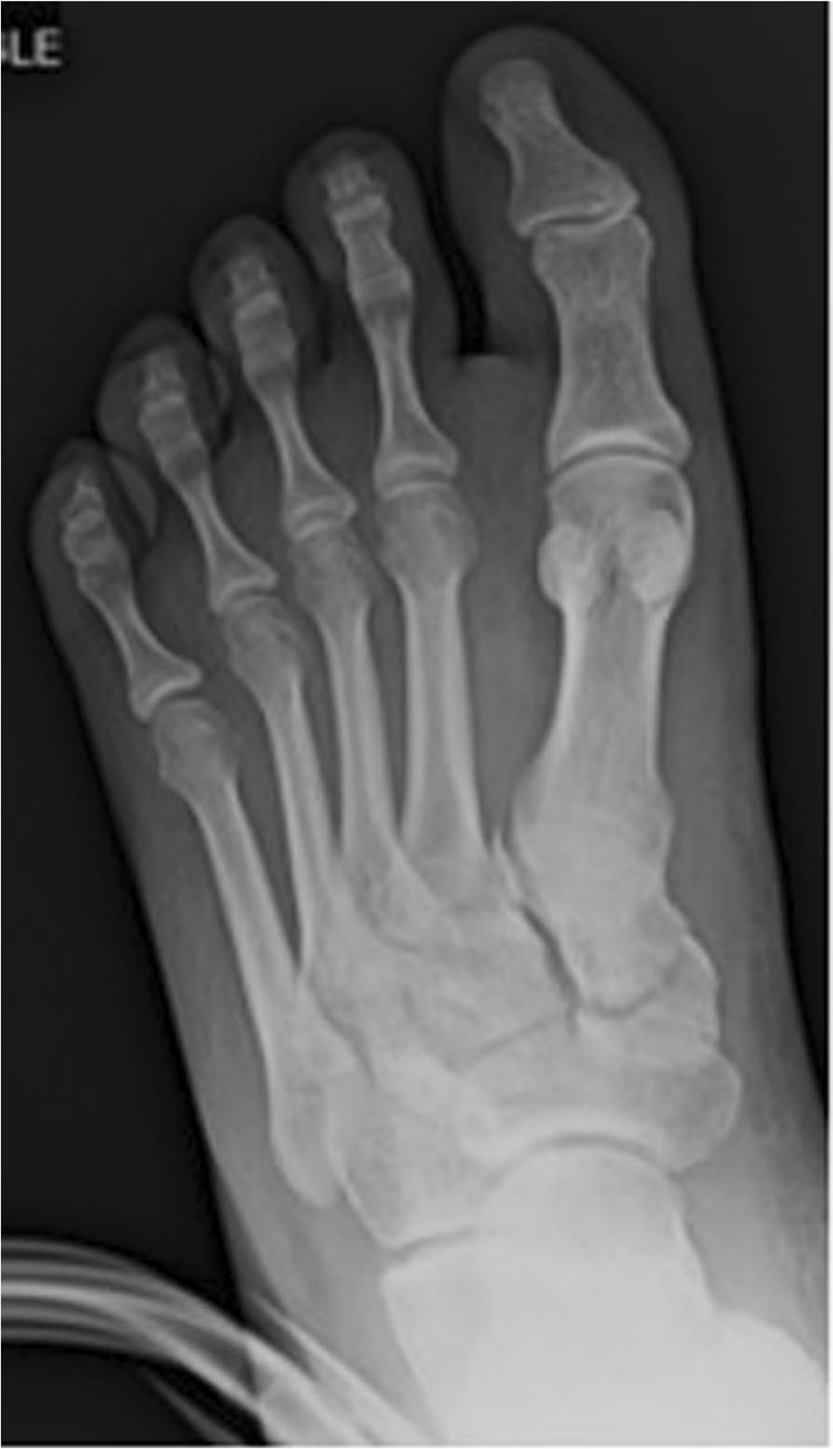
Anteroposterior radiograph of left foot demonstrating “fleck” sign pathognomonic for Lisfranc joint disruption
